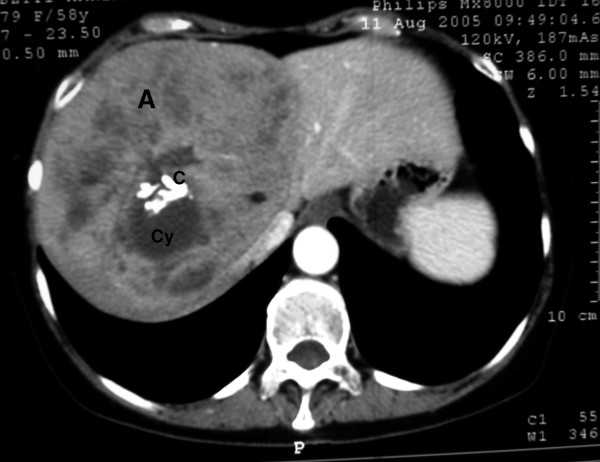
Contrast-enhanced CT scan. A: adenoma; RHV: right hepatic vein; MHV: middle hepatic vein (portal venous phase).
